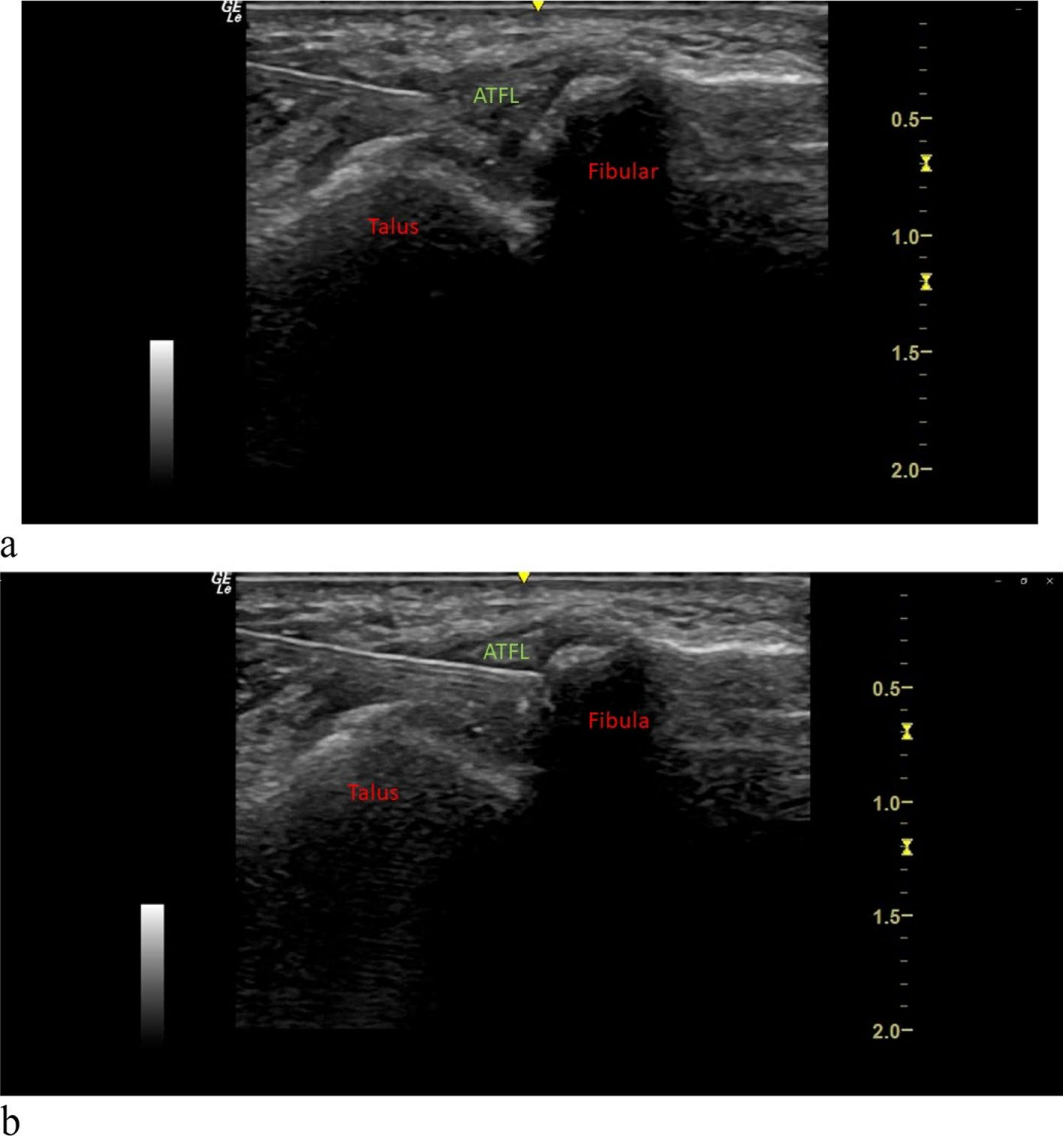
a Injection of anterior talofibular ligament, in-plane approach under ultrasound guidance (talus end). b Injection of anterior talofibular ligament, in-plane approach under ultrasound guidance (fibula end)
